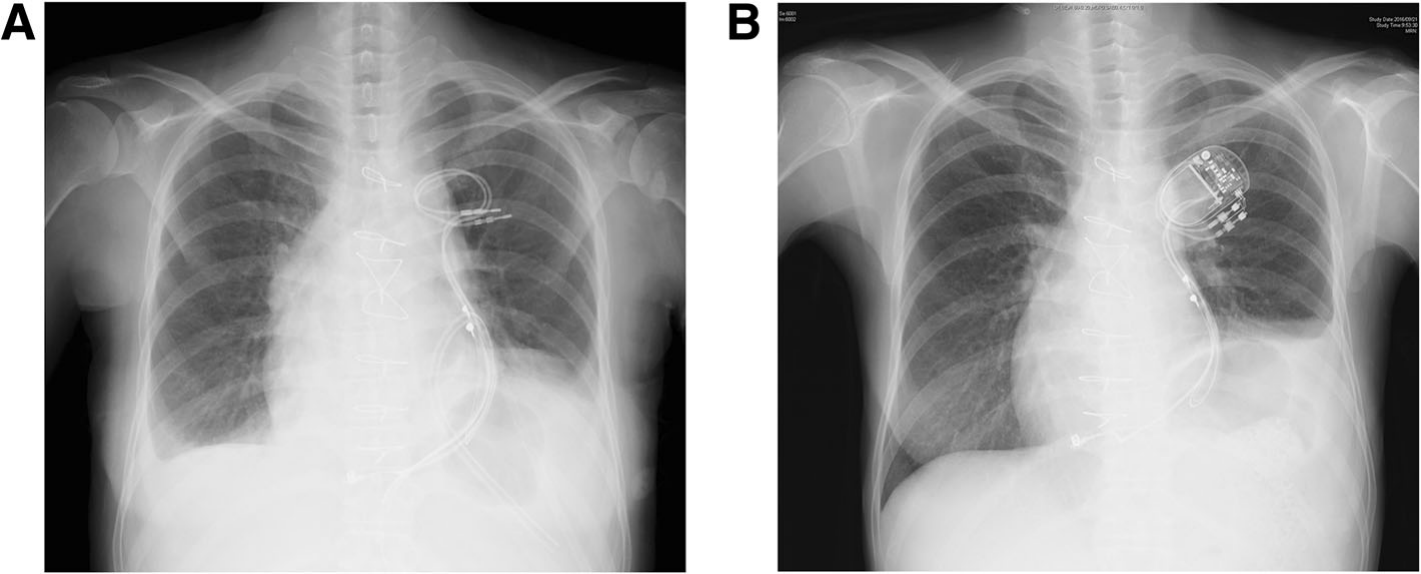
The chest X-ray images a immediately after the TCPC conversion on the fourth postoperative day and b on the current admission, showing significant elevation of the left dome of the diaphragm, suggesting left hemidiaphragmatic paralysis
